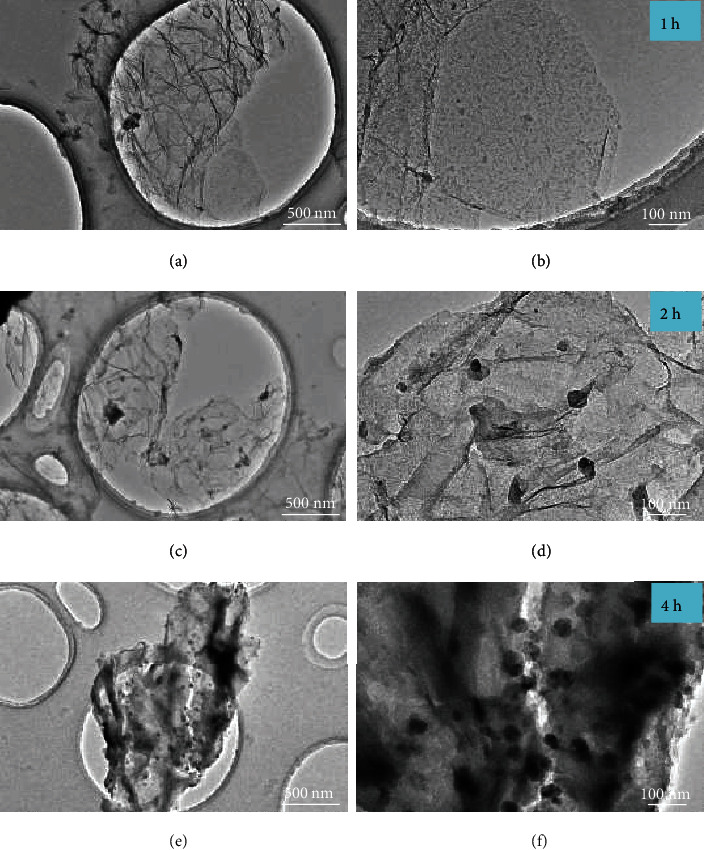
TEM images of NP-TiH_2_@G with the ultrasonic time of 1 h (a, b), 2 h (c, d), and 4 h (e, f).
